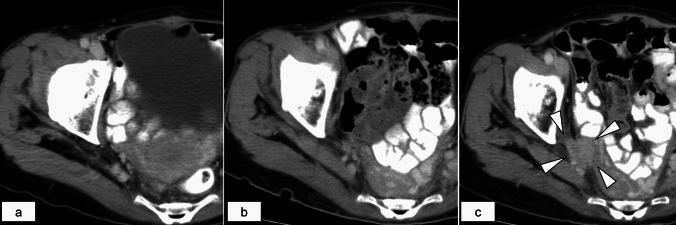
A representative case of lateral pelvic recurrence after lateral pelvic lymph node dissection. Computed tomography images obtained preoperatively (a), 3 months after surgery (b), and 2 years after surgery (c) are shown. The white triangles indicate recurrent nodules
